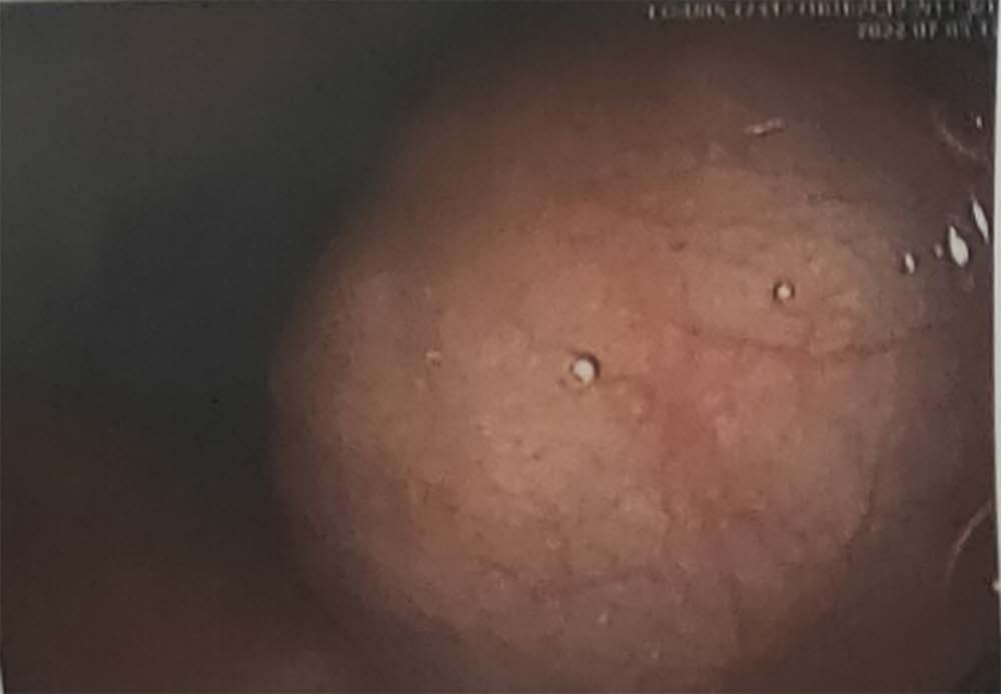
An electron circumferential ultrasound endoscopy showed a hemispherical bulge with a smooth surface 18 to 23 cm from the incisor; the bulge originated from the intrinsic muscular layer and showed a heterogeneous mixed moderate ultrasound with a little blood flow signal and blue-green elastography in 1 of the sections measuring approximately 4 cm × 3 cm.
